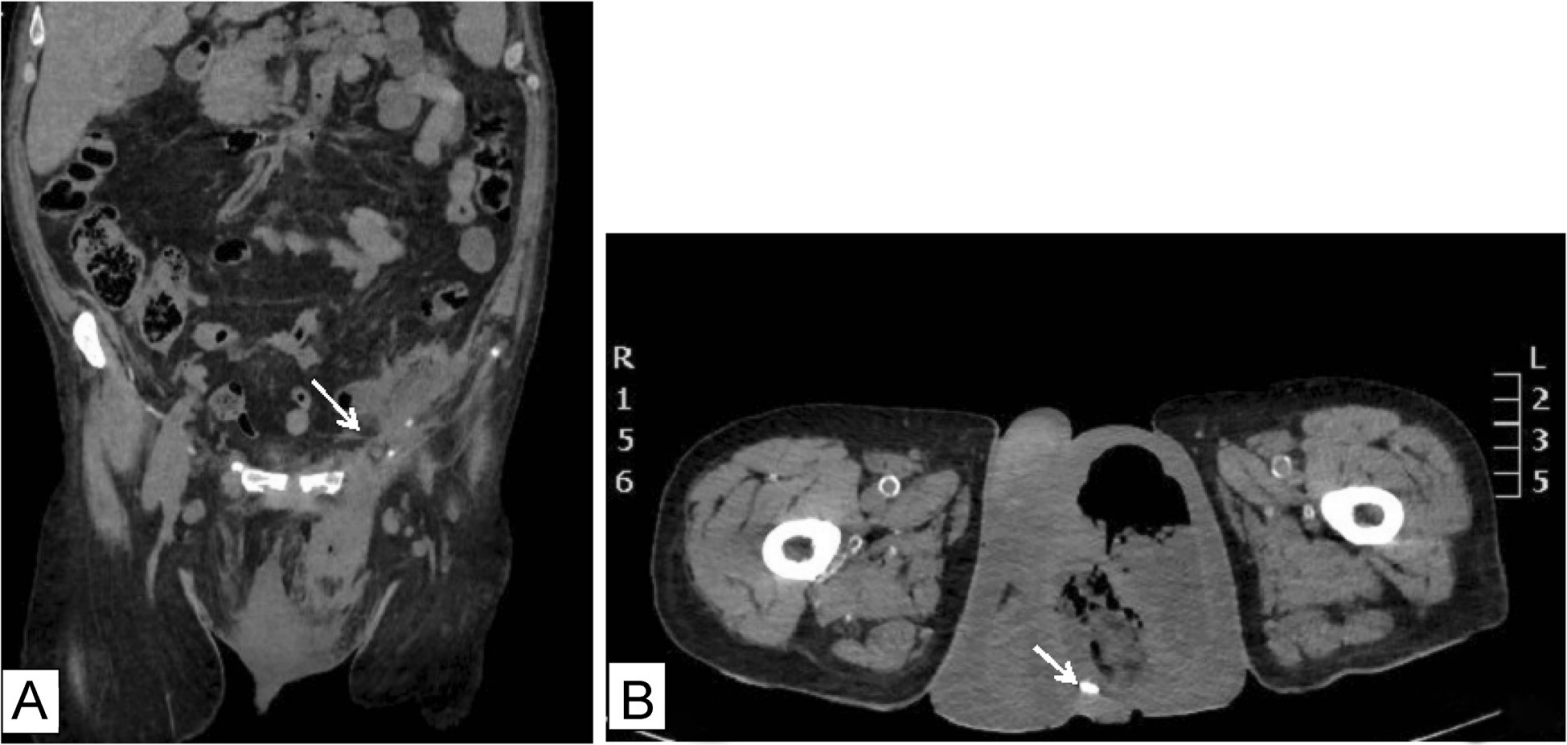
a: Initial CT scan showing sepsis, communicating between scrotum and the preperitoneal space, through the internal ring, adjacent to a sigmoid colon segment(arrow). b: Watermelon seed (foreign body) seen in the left hemi-scrotum(arrow)
